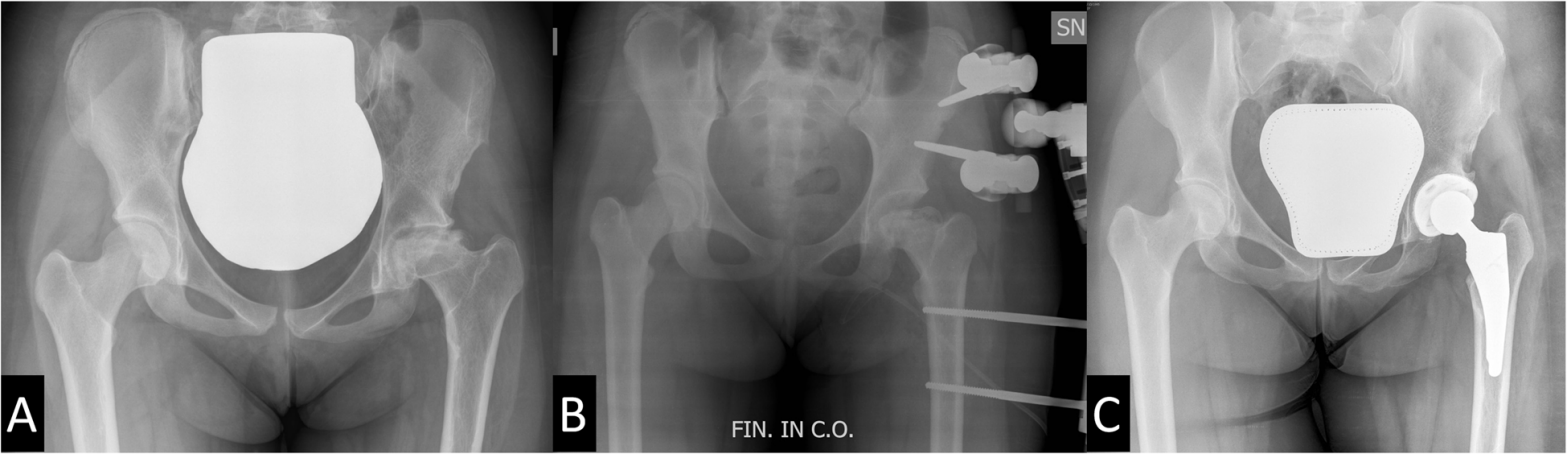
a Plain radiographs of a 19-year-old female with LCPD (patient 7). b Arthrodiatasis of the left hip with external fixator. c Postoperative radiograph after left THA using posterolateral approach
